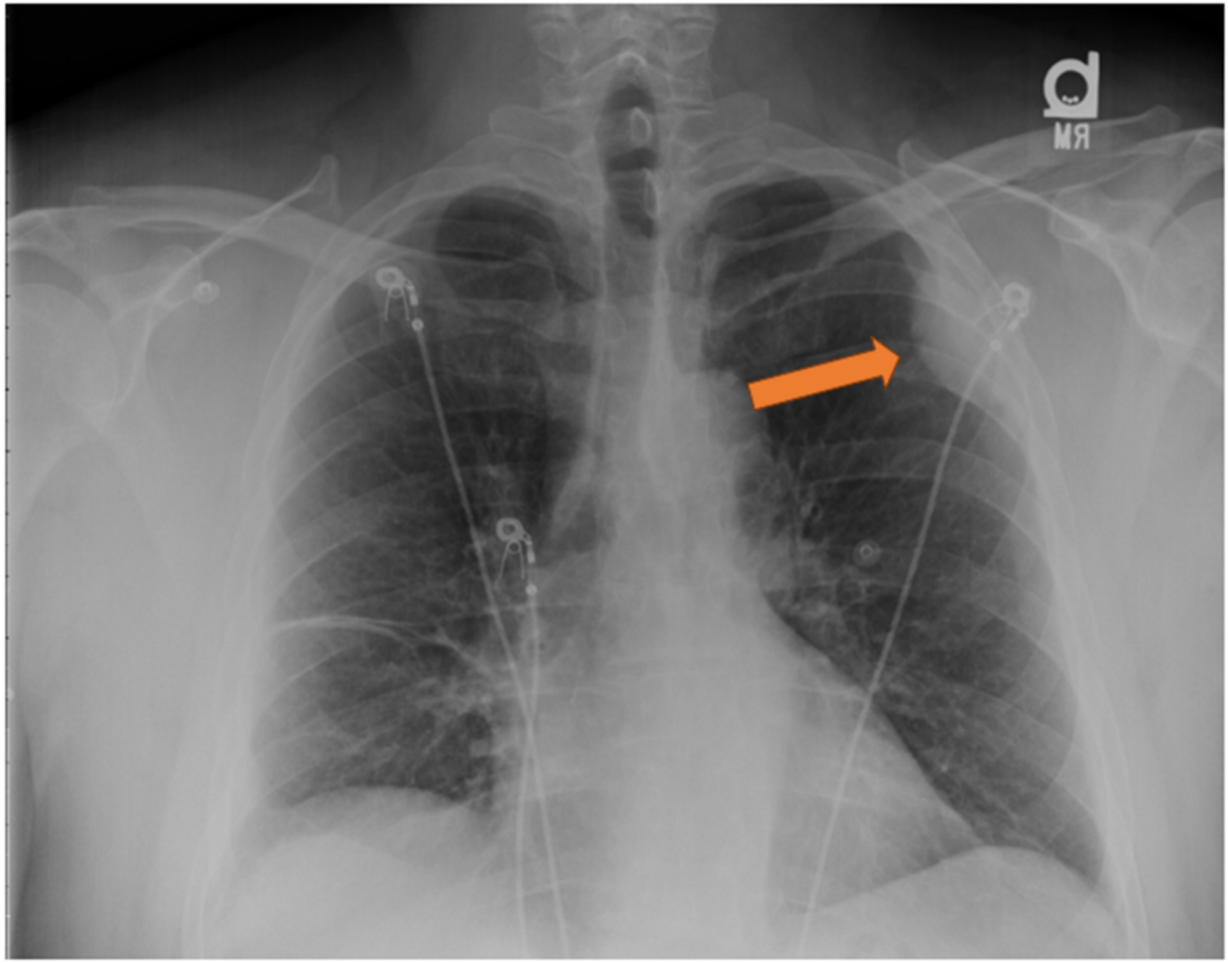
Chest X-ray, posteroanterior view Chest X-ray (posteroanterior view) showing peripheral opacity in the left upper lobe (orange arrow).
